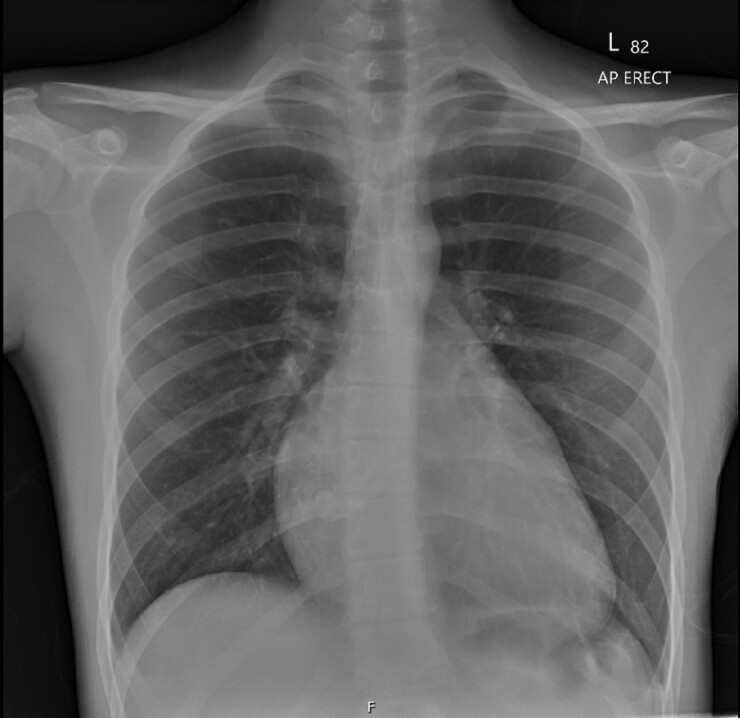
Antero-posterior chest X-ray demonstrating globular cardiomegaly.
